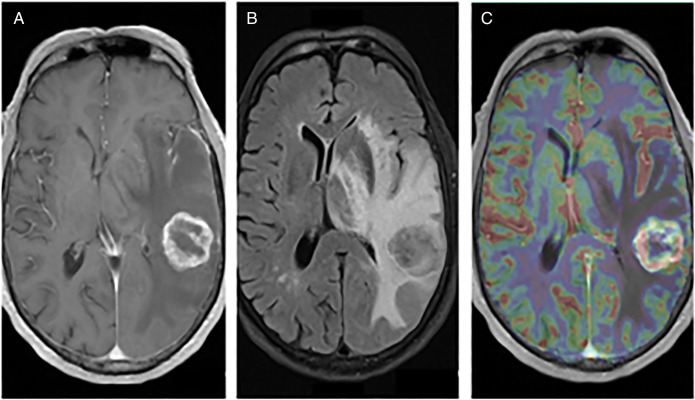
Preop imaging of L parietal lobe mass. (A) T1 axial 1 GD, (B) T2 FLAIR, and (C) T1 OLEA. FLAIR, fluid-attenuated inversion recovery.
